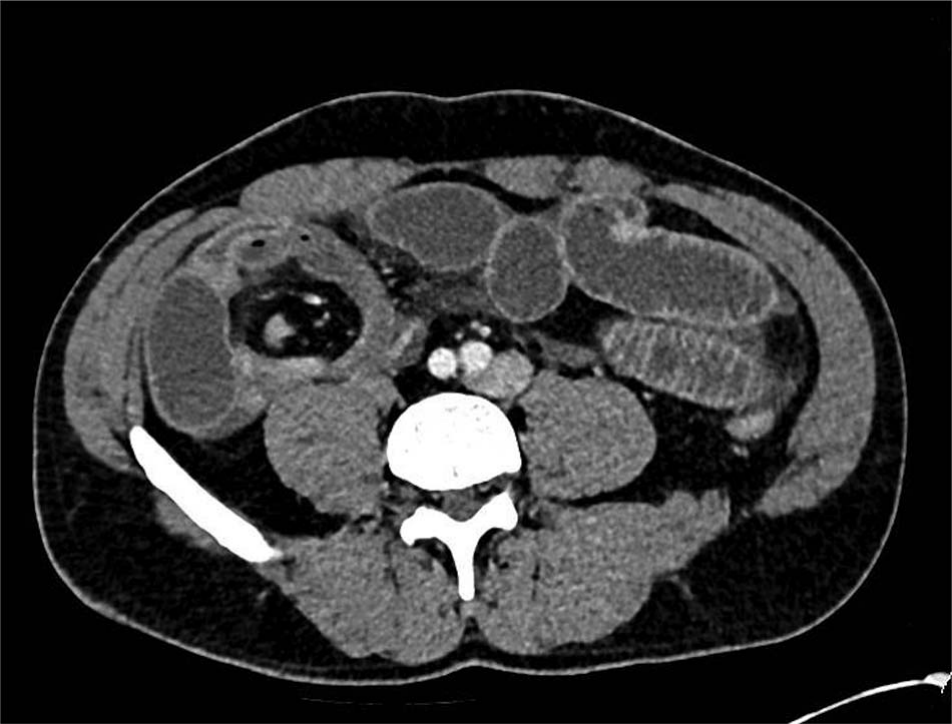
CT showing abnormality in right iliac fossa with dilated bowel loops.
